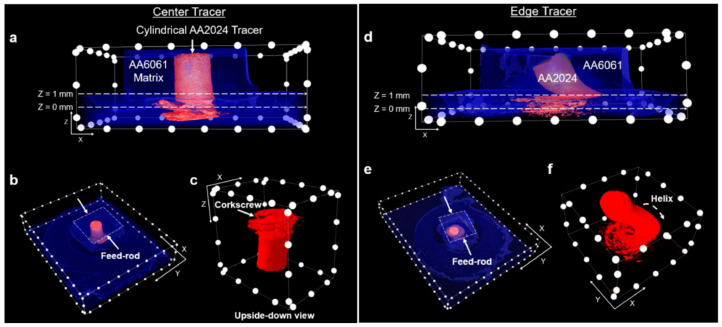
CT results following the initial feeding of the feedstock for the (a–c) center tracer and (d–f) edge tracer. The AA2024 tracer is highlighted in red while the encompassing AA6061 matrix is shown in blue or a transparent state. The spacing between each two white dots is 2.5 mm [16].
